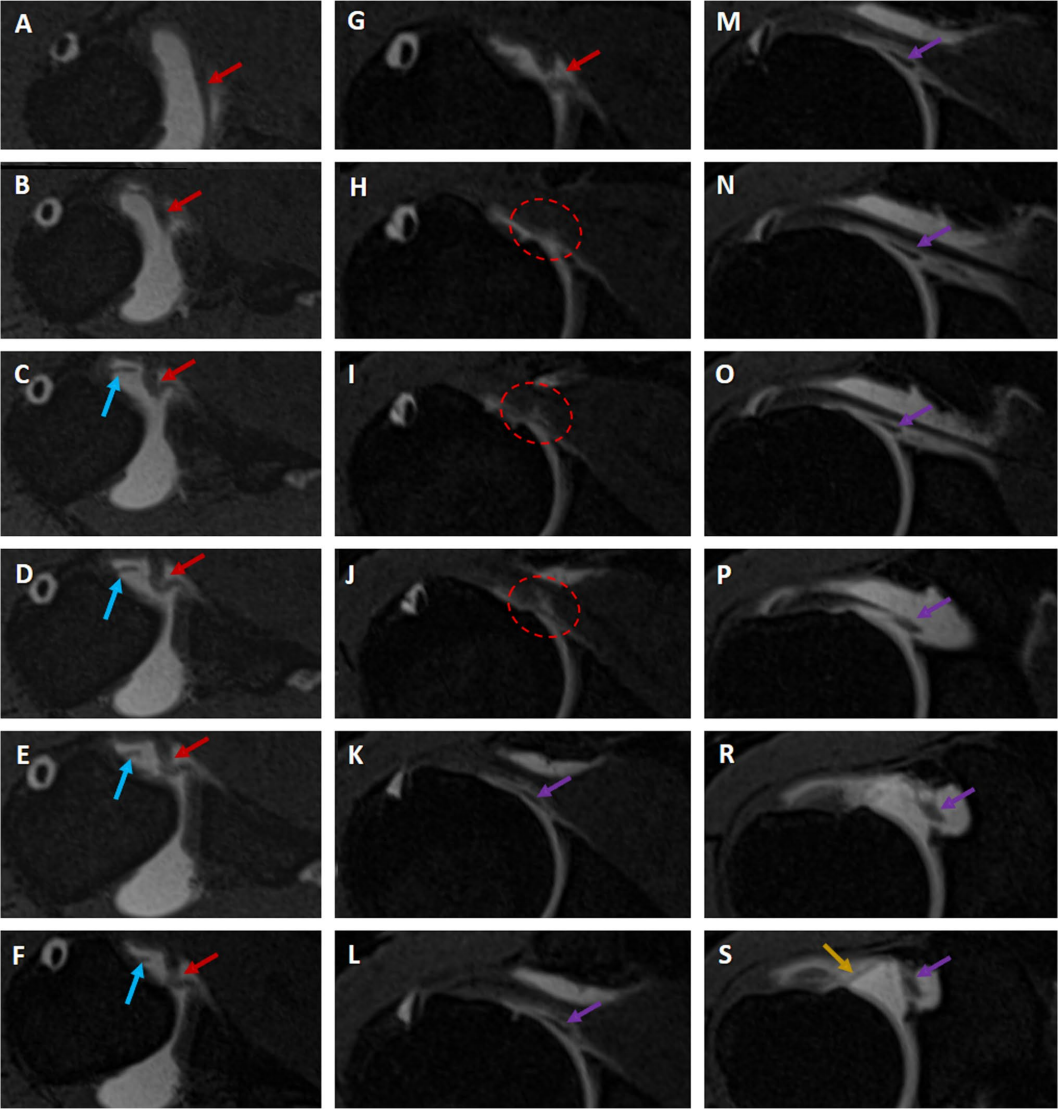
Consecutive axial A–S VIBE T1-weighted MR arthrograms demonstrate SpiGHL (red arrows) conjugating (circle in frames H, I, and J) with MGHL (purple arrows) and other ligamentous structures such as SGHL (yellow arrow) and AB-IGHL (blue arrows)
